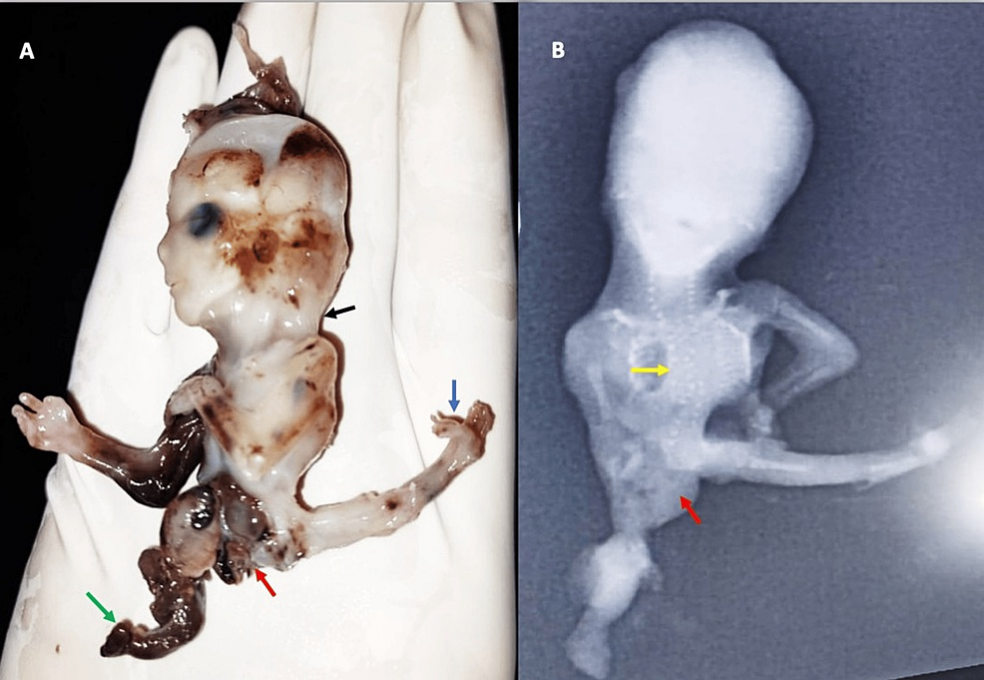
Post-delivery image (A) showing eviscerated abdominal organs without membranous covering (red arrow), nuchal thickening (black arrow), a limb-like soft tissue adherent to the externalized abdominal organs on the right side, hypoplastic right lower limb (green arrow), and a malrotated left lower limb with deformed foot (blue arrow). On post-natal X-ray imaging (B), distorted abdominal contour was noted with ill-defined opacities. Soft tissue opacification (red arrow) was seen along the right lower limb; however, no defined ossified bones in any of the three segments were noted compared to the left side. Furthermore, a mild kyphoscoliotic curvature of the spine with unossified vertebral bodies in the cervical and lumbosacral region (yellow arrow) was also noted.
